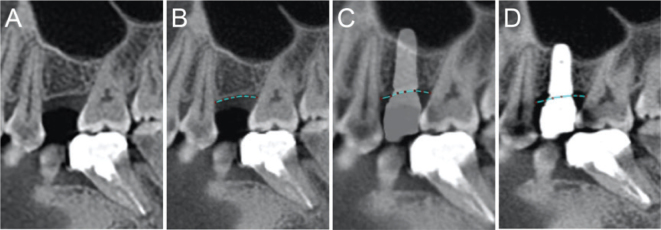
Comparison analysis of CBCT images before implantation and 3 years after implantation, with Figures 2A and 2B representing pre-implantation CBCT images, and Figures 2C and 2D representing CBCT images 3 years after implant loading. The blue dashed line in Figures 2B, 2C, and 2D indicates the alveolar bone margin before implantation.
